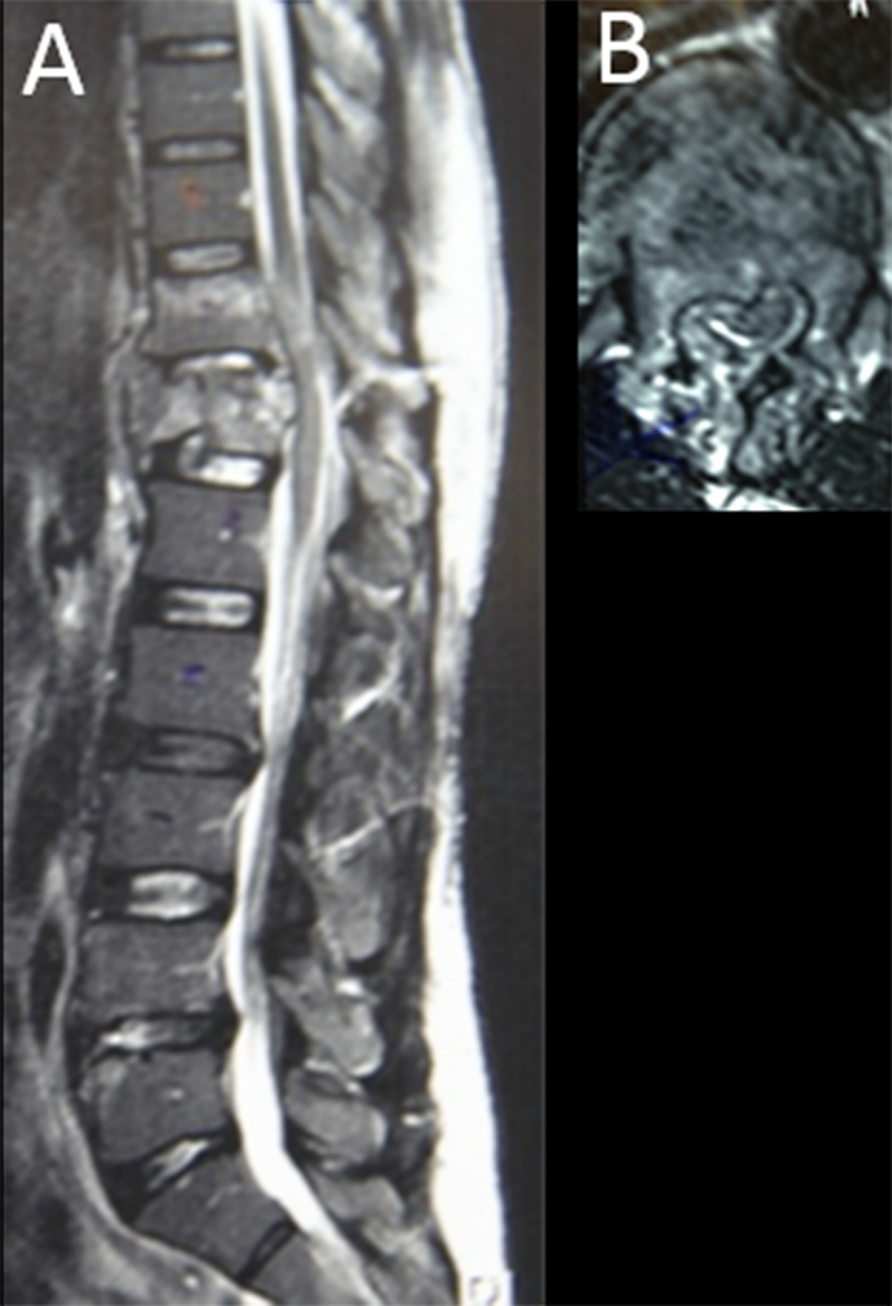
Burst Fracture at T12 (a) Sagittal and (b) axial MRI scan of the patient’s stable burst fracture at T12.
